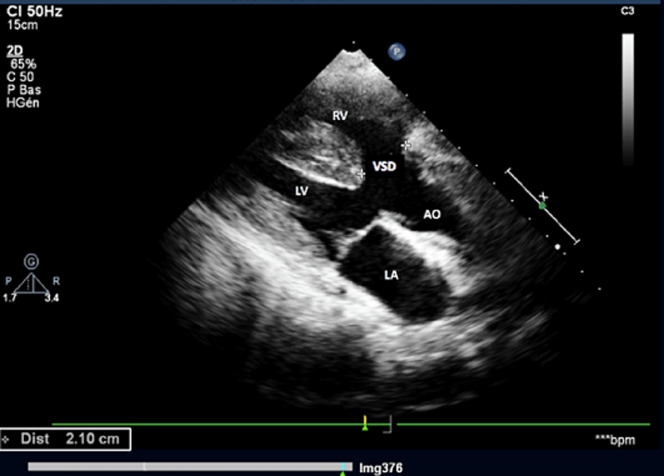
parasternal long-axis echocardiographic view showing ascending aorta overriding the interventricular septum
